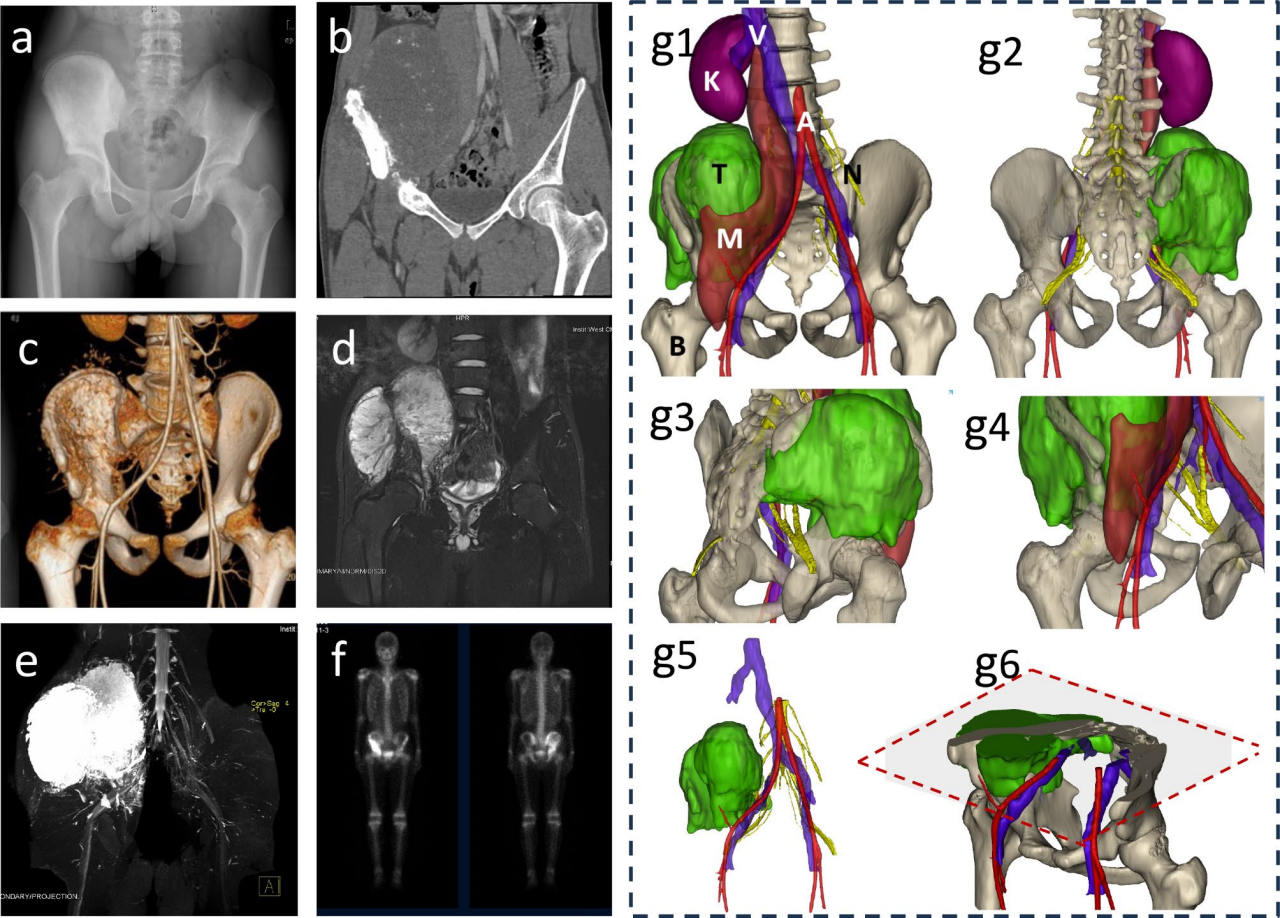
The 3DMMI fusion image of a typical pelvic bone tumor case: (a) preoperative pelvic X-ray image, (b) Pelvic 3D CT, (c) 3D-CT angiography, (d) contrast-enhanced MRI displaying the tumor and its soft tissue boundaries, (e) diffusion tensor imaging of the sacral plexus, and (f) whole-body nuclear medicine bone scanning (ECT). (g1) The anterolateral view displays a lesion extending from the periacetabulum to the level of the kidney, involving the sacrum. The right external iliac vessels and ureter remain unaffected due to the protection provided by the iliacus muscle and psoas major muscle. Abbreviations: K, kidney; M, muscle; N, nerve; T, tumor; V, vein; A, artery; B, bone. (g2-3) The posterior perspective images using pelvic transparency techniques, allowing clear visualization of internal anatomical structures within the pelvis. A significant tumor is observed, with penetration of the second and third sacral nerves, indicating neural encroachment. (g4) The 3D reconstructed image displays the relationship between the anterior thigh blood vessels and the tumor. (g5) Hide other non-interest areas and observe the relationship between the tumor and blood vessels and nerves. (g6) Observe the relationship between the tumor and other surrounding structures through cross-sectional views
